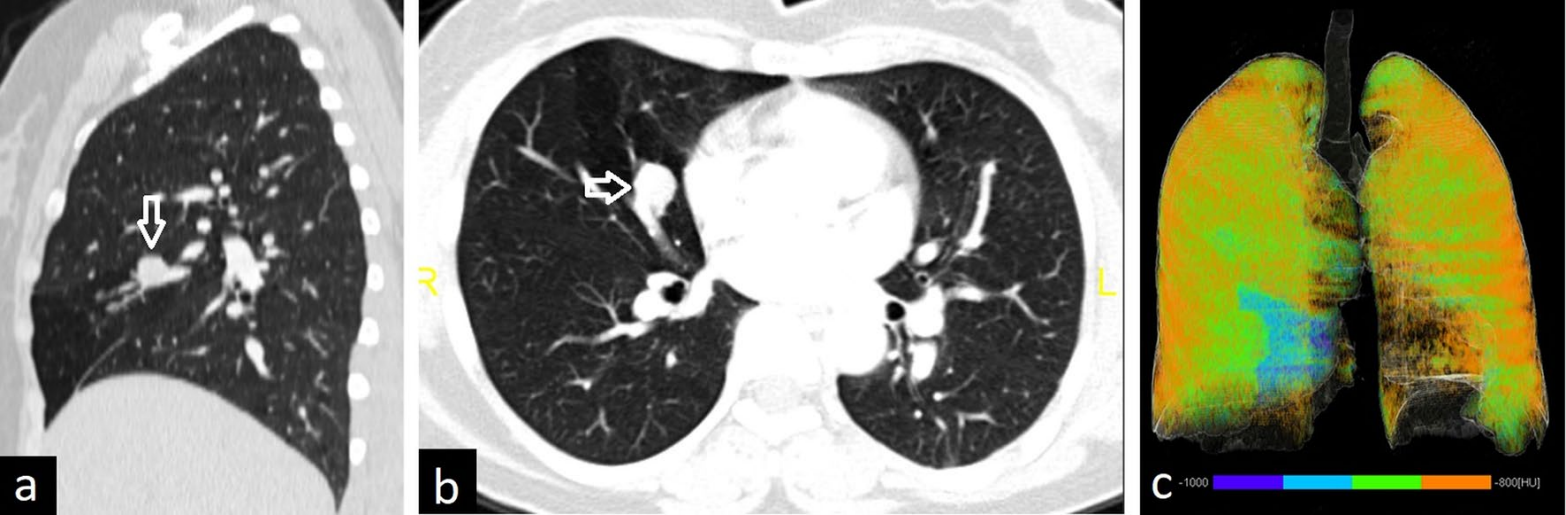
Bronchial carcinoid mimicking bronchial atresia. Sagittal (a), axial (b), and quantitative 3D (c) CT images show that a bronchial carcinoid (arrows) can mimic bronchial atresia because of air-trapping in the distal segment of the mass
